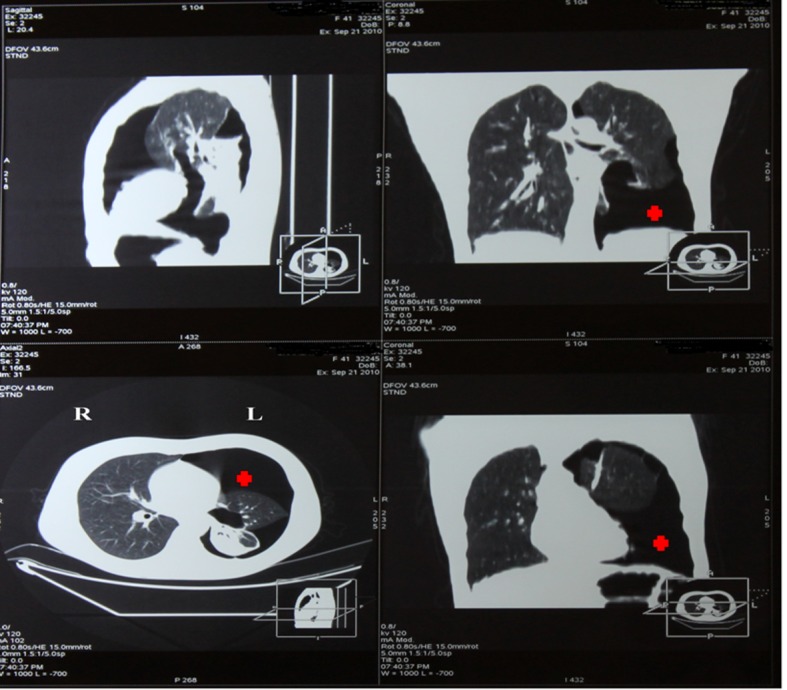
HRCT with big pneumothorax in the left lower hemithorax (red cross).
